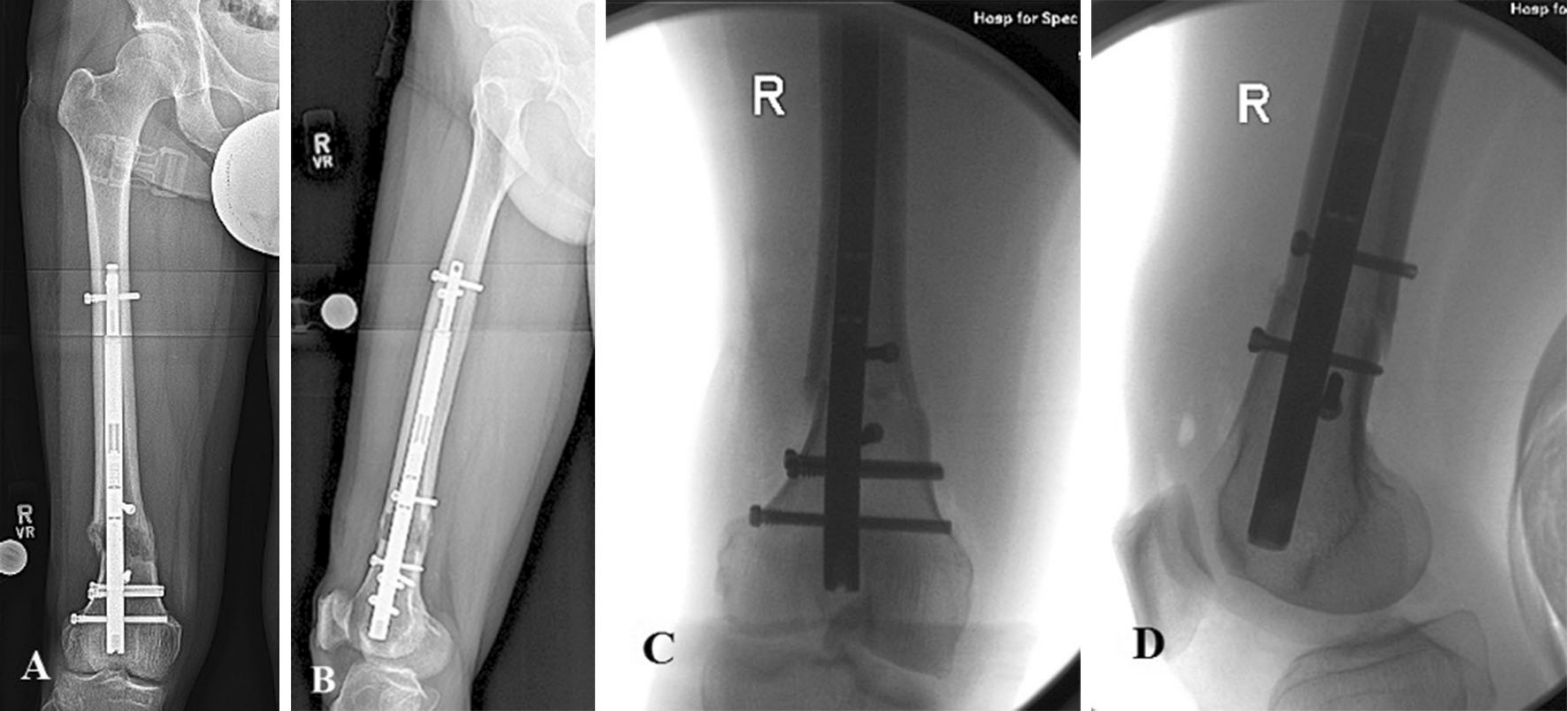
Retrograde femoral nail with distal femoral osteotomy. Anteroposterior radiograph (a) and intraoperative fluoroscopic anteroposterior view (b) show two medial blocking screws, one in proximal fragment and one in the distal fragment near the osteotomy site used to prevent varus angulation of the proximal and distal fragments, respectively. Lateral radiograph (c) and the intraoperative fluoroscopic lateral view (d) show one posterior blocking screw in the distal fragment near the osteotomy site used to prevent procurvatum deformity
